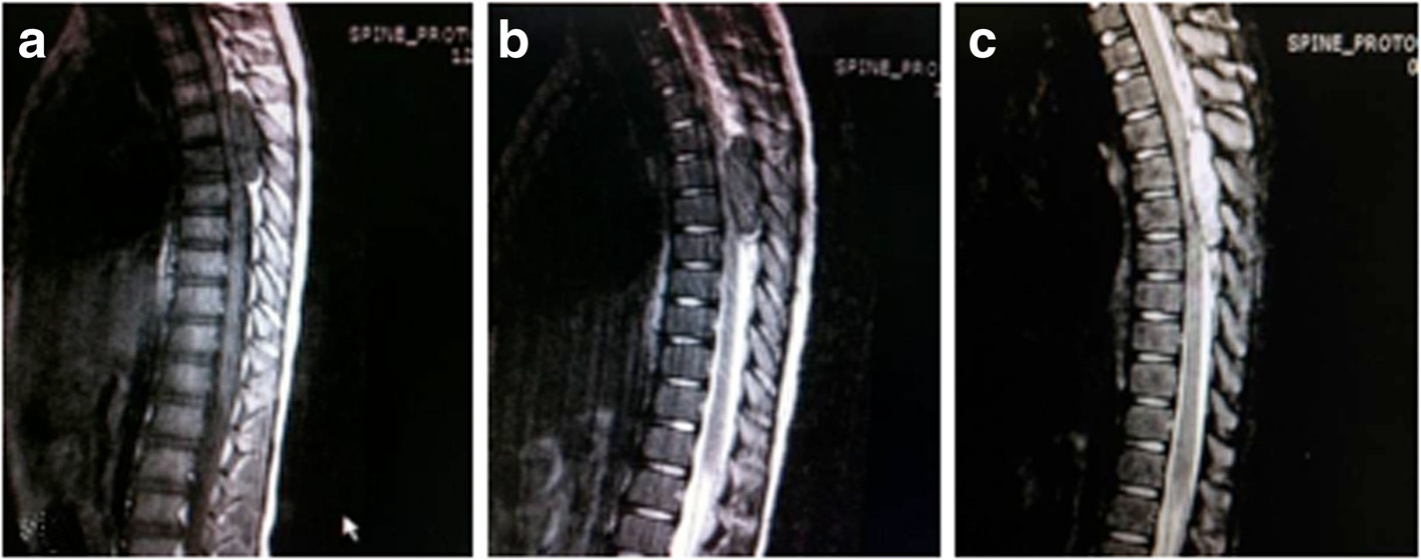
Preoperative sagittal magnetic resonance imaging. a. Preoperative sagittal T1-weighted magnetic resonance imaging of the dorsal spine showing extradural mass in posterior aspect of dorsal canal approximately 1.0 × 1.4 × 4.0cm in size along T4 to T7 levels with hypointense signal. b. Preoperative sagittal T2-weighted (T2W) magnetic resonance imaging showing extradural mass with intermediate intense signal. c. Preoperative sagittal post-contrast T2W showed intense homogeneity with significant enhancement.
